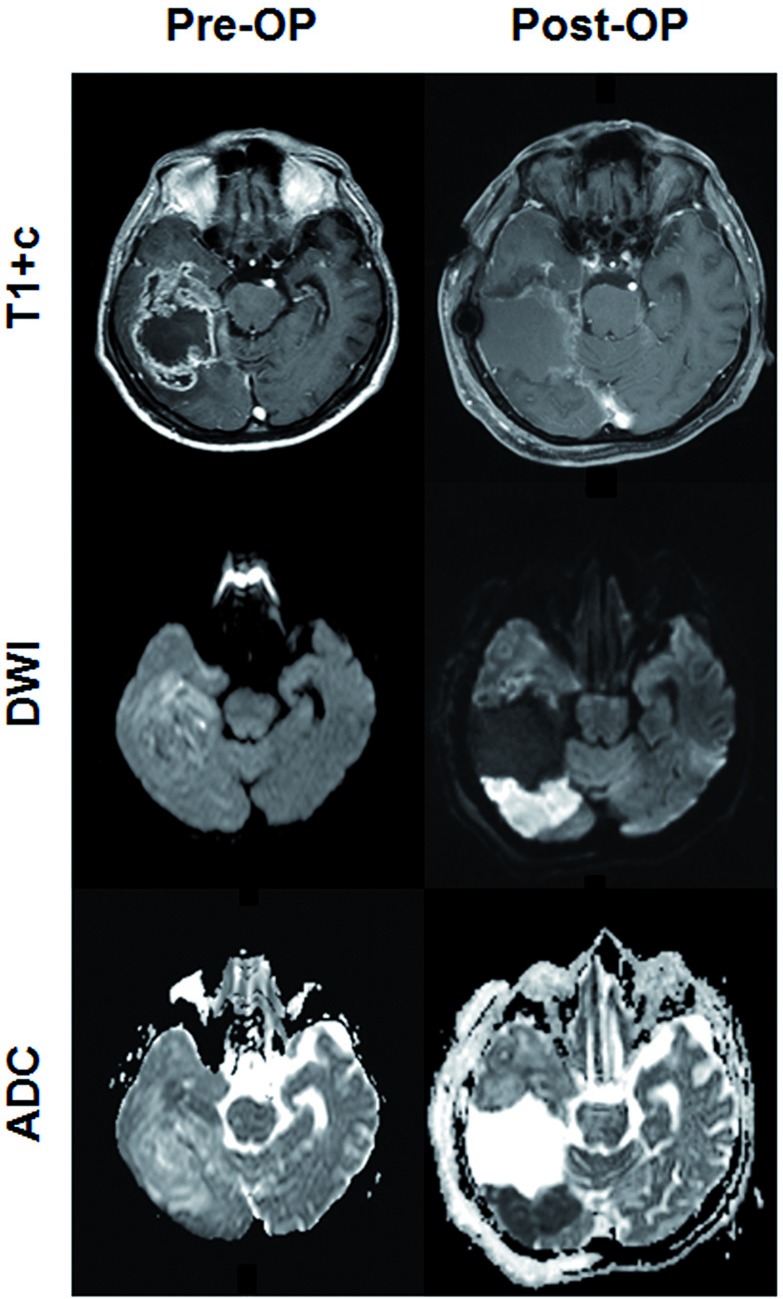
Perioperative ischemia Representative pre- and postoperative (< 72h after surgery) MRI scans from a patient with perioperative ischemia ARE shown.
